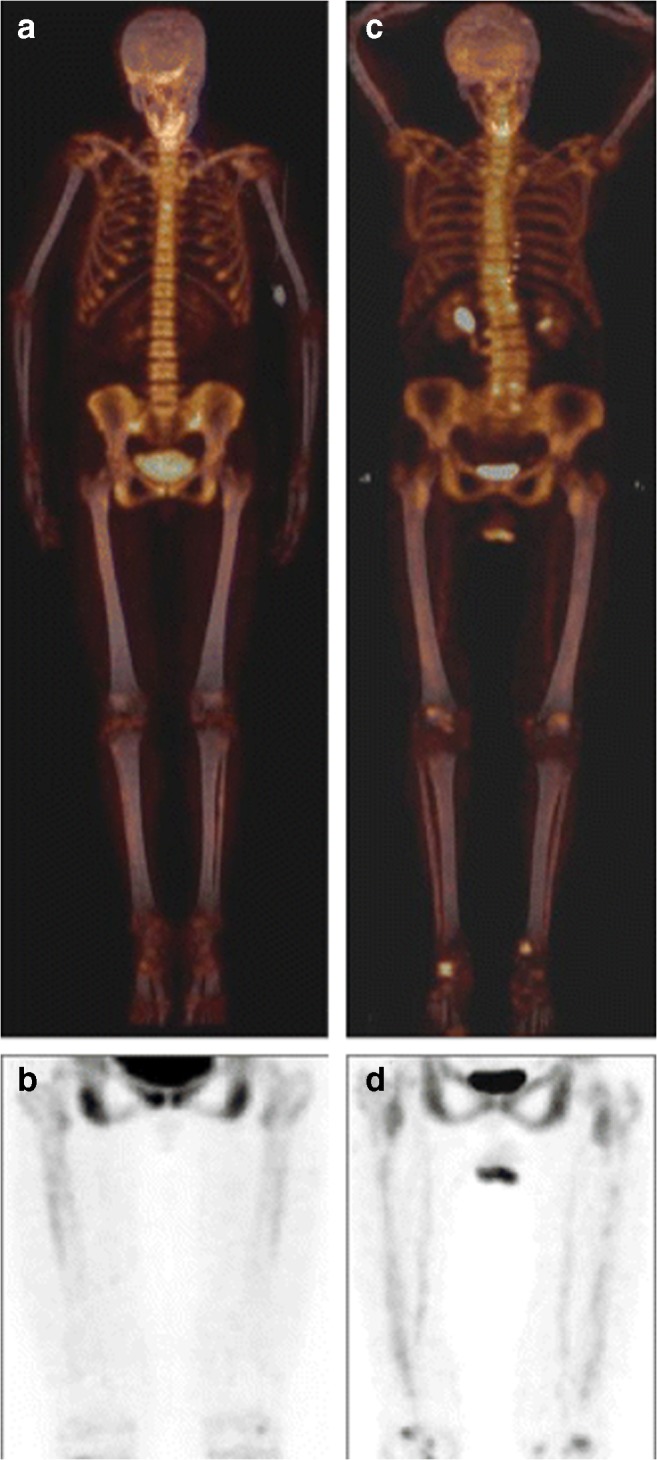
Arterial mineral deposition increases with age, while regional bone metabolism decreases. Coronal fused 18F-sodium fluoride PET/CT (a) and non-fused PET of the thighs (b) of a 29-year-old woman with low arterial mineral deposition (TBR 1.44) and high bone metabolism (SUVmean 8.6). Coronal fused 18F-sodium fluoride PET/CT (c) and non-fused PET of the thighs (d) of a 83-year-old woman showing high arterial mineral deposition (TBR 2.18) and low bone metabolism (SUVmean 6.7) (reproduced by permission from reference 25)
